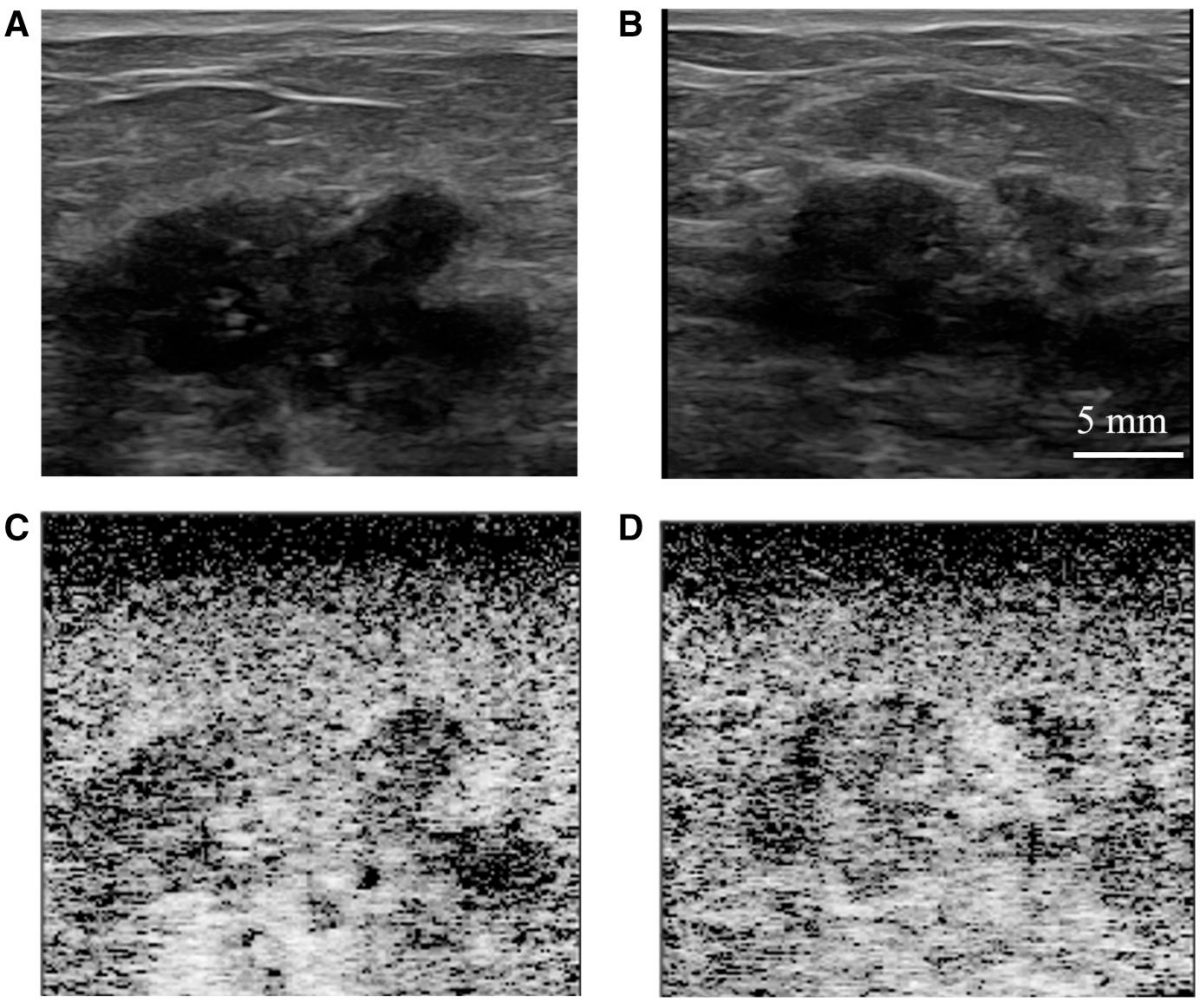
A 69-year-old woman was called back from screening mammogram for a left breast focal asymmetry. Pairs of B-mode ultrasound static images show a 3.1 cm suspicious mass seen in the (A) antiradial and (B) radial projections at 1.5 cm depth from the ultrasound transducer. (C) Antiradial and (D) radial SLSC images of the same finding show similarly coherent lesion content relative to the surrounding tissue. Five out of 6 radiologists characterized the finding as solid based on B-mode and SLSC ultrasound images, 1 radiologist characterized the finding as mixed based on B-mode and SLSC ultrasound images, and the 6 radiologists each drew regions of interest that resulted in gCNR values ranging 0.14 to 0.36 for the SLSC image, which categorizes the finding as solid. The mass was biopsied, and the pathology report revealed an invasive ductal carcinoma, moderately differentiated.
